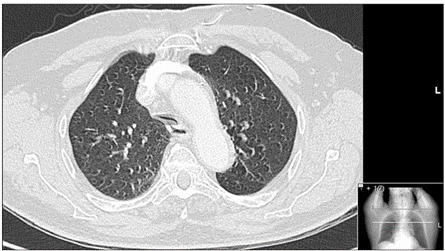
Chest computed tomography showing lung emphysema and cross-sectional collapse of the trachea.
